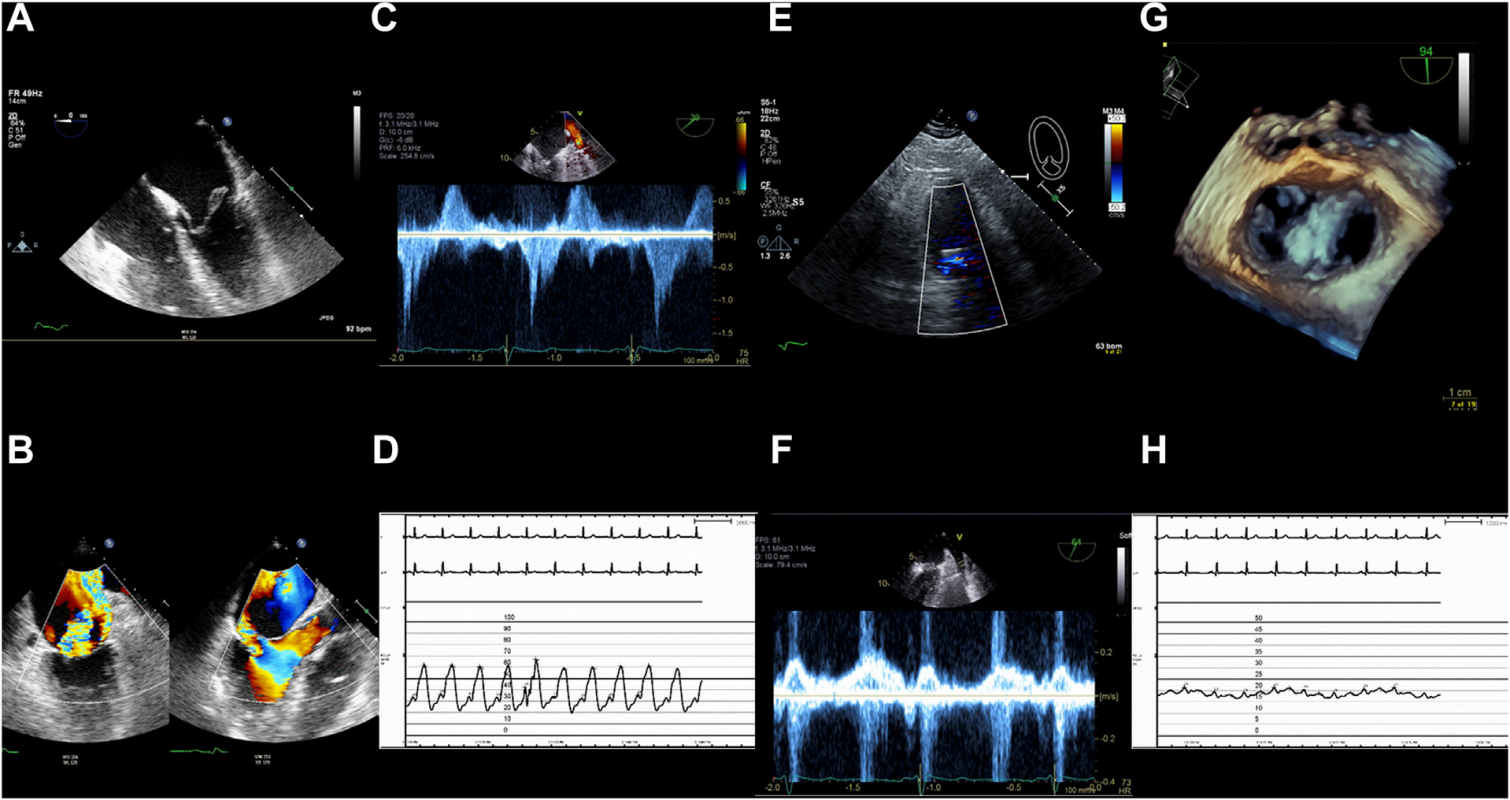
Case 1 summary. (A) TEE: complete rupture of the anterolateral papillary muscle. (B) TEE: severe mitral regurgitation across A2-P2. (C) TEE: pulmonary vein systolic flow reversal. (D) Elevated left atrial V wave pressure of 62 mm Hg. (E) Transthoracic echocardiogram: postprocedural mild mitral regurgitation. (F) TEE: postprocedural normalized pulmonary vein systolic flow. (G) TEE: postprocedural multiplanar reconstruction. (H) Reduced left atrial V wave pressure of 20 mm Hg. TEE, transesophageal echocardiogram.
